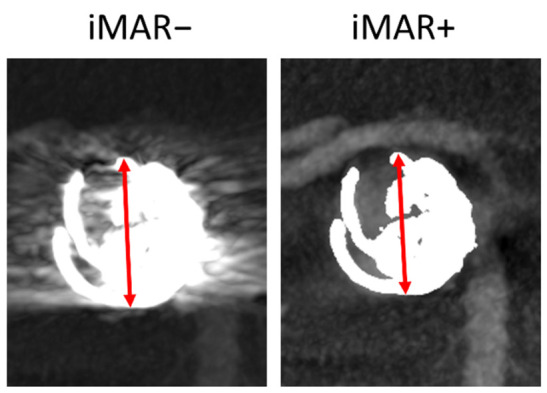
Exemplary CPD measurement in iMAR−/+ FD-CTA images. CPD is indicated by the red arrow.
